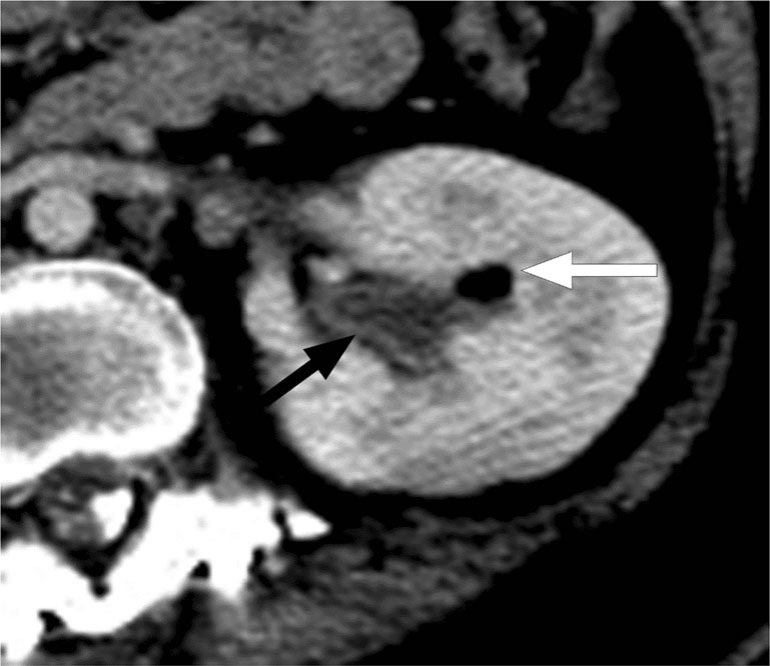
Pyelitis. Axial image from contrast-enhanced CT shows air within the left renal collecting system (*white arrow*). There is uroepithelial thickening and enhancement (*black arrow*)
